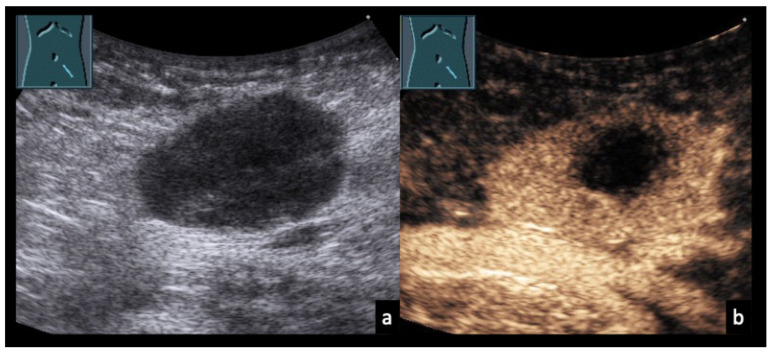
49-year-old male patient with grade 1 follicular lymphoma prior to therapy. (a) B-mode ultrasound shows a predominantly hypoechoic inguinal lymph node. (b) On CEUS, there is central absence of enhancement, most consistent with necrotic transformation.
